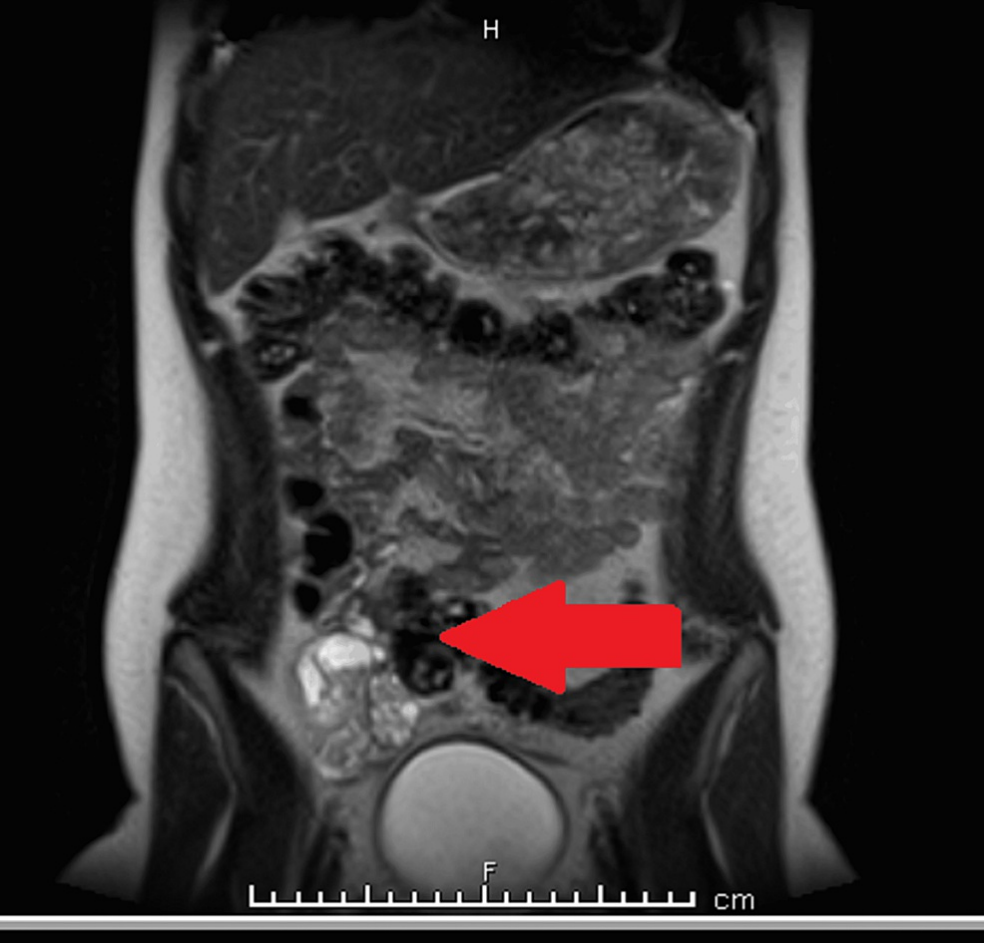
MRI showing right salpingitis
